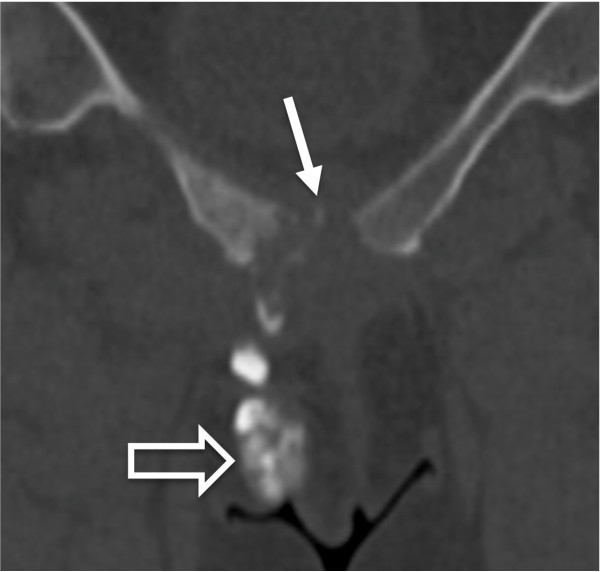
Coronal reformats of computed tomography examination, obtained after direct injection of contrast material into the pubic symphysis (arrow), shows continuity of the arthritis with the collection prolonging to the labia (open arrow).
